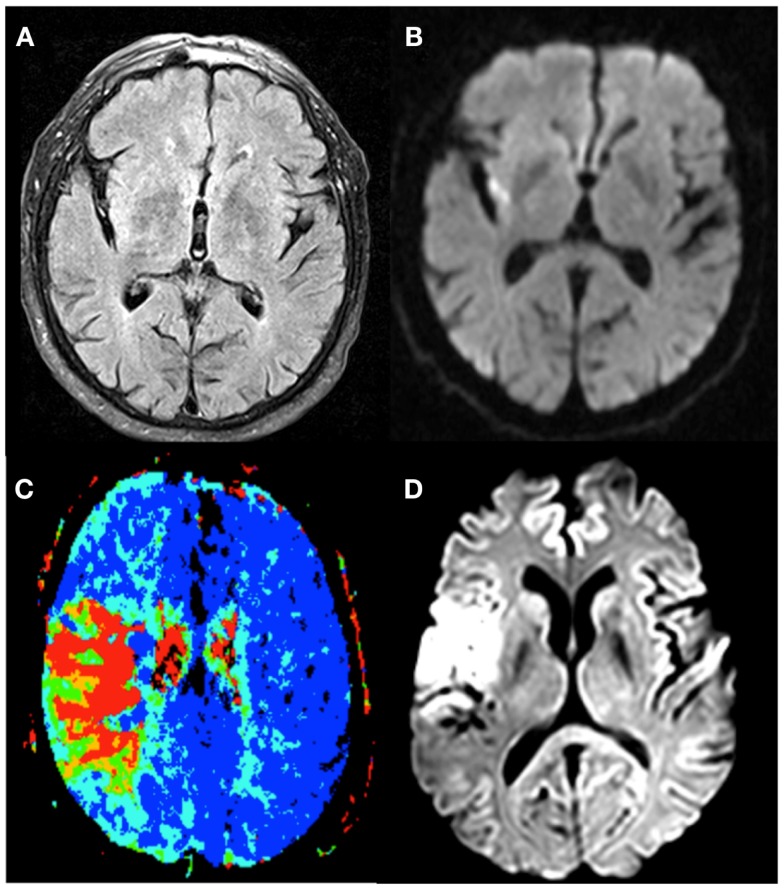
The different imaging techniques used to select stroke-patients who would benefit from therapy. Patient 1 exhibits no FLAIR-lesion (A) and a clear DWI-lesion (B), the so-called DWI-FLAIR mismatch pattern. Patient 2 has a PWI/DWI mismatch on imaging, with (C) representing the lesion on Tmax, and (D) the corresponding diffusion lesion.
